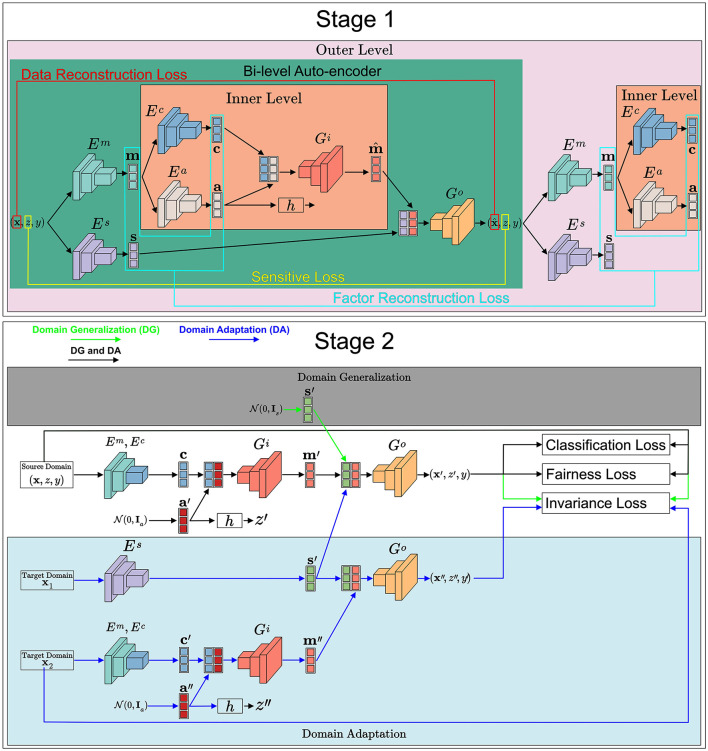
An overview of our FFDGUA framework. The learned transformation model from Stage 1 generates augmented samples to train a robust classifier. For DG, it synthesizes arbitrary unseen domains by sampling style factors from a prior Gaussian distribution. For UDA, it explicitly extracts exact style factors from unlabeled target domain samples. Finally, the framework optimizes the classifier using a joint objective of losses.
